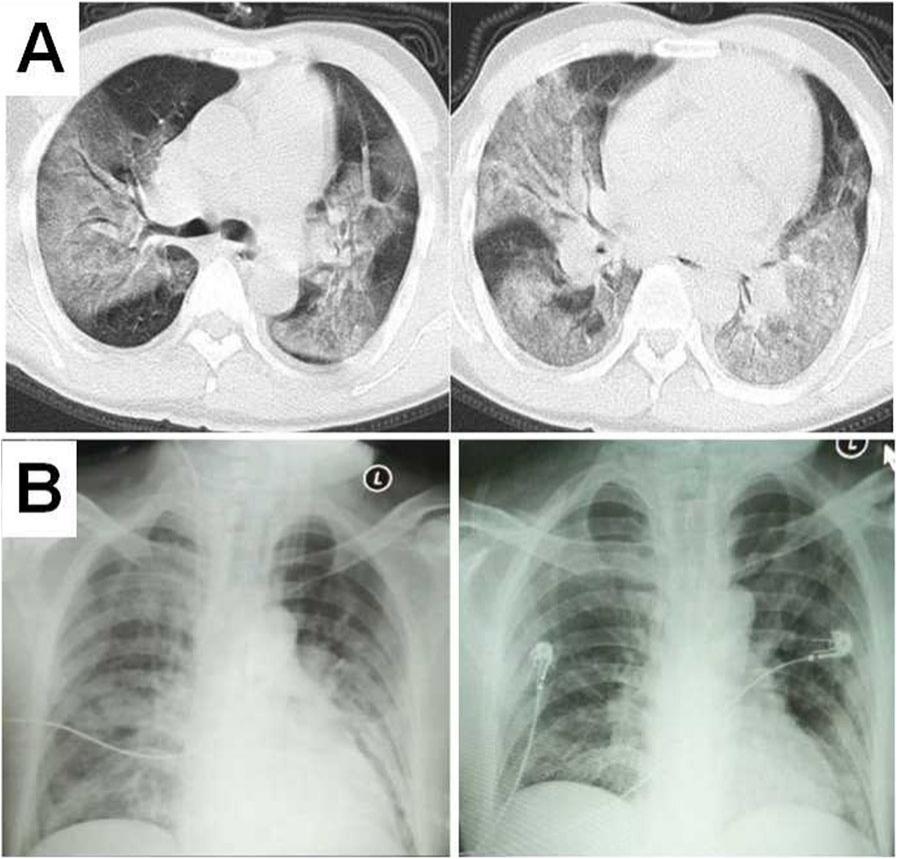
Computed tomography scan on admission to ICU showing extensive bilateral ground-glass opacities and consolidations(a). The chest X-ray examination on admission to ICU showing bilateral diffuse interstitial infiltrates (B, left). The chest X-ray examination on 8 days after admission to the ICU revealing the consolidative opacities in the left lower lung zones (b, right)
